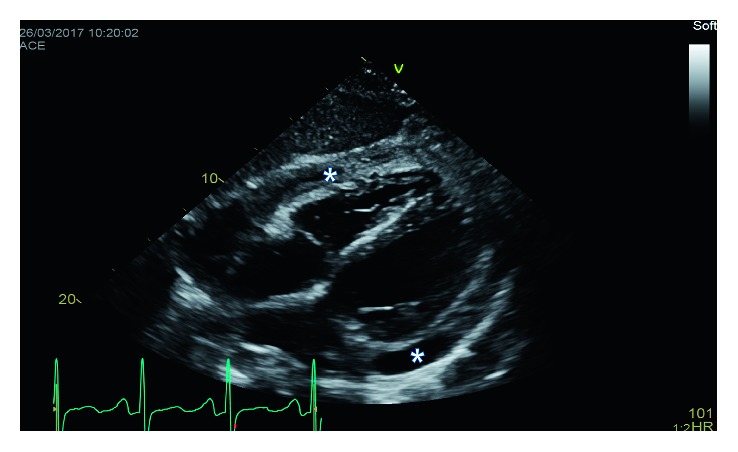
Transthoracic echocardiogram subcostal view (pericardial effusion denoted by ∗).
